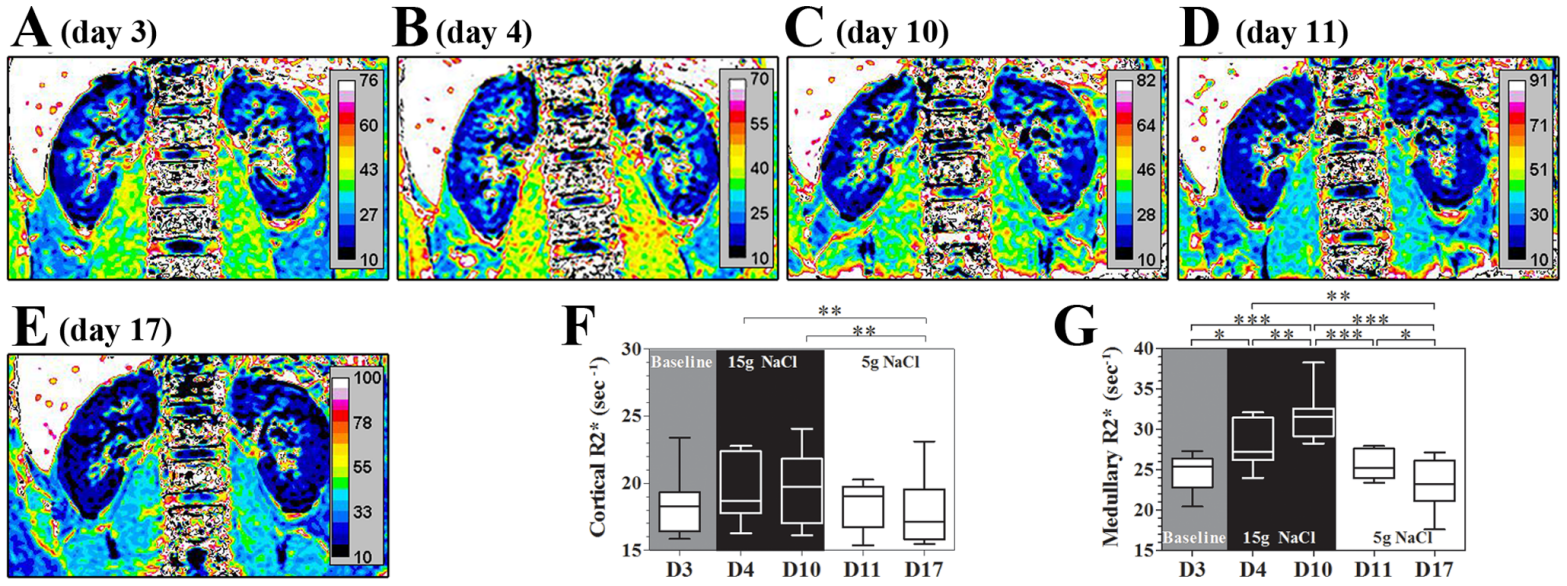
Renal blood oxygen level dependent-magnetic resonance imaging (BOLD-MRI) during dietary intervention. Figures A to E show the representative changes of BOLD-MRI images from one participant during dietary intervention on day 3, day 4, day 10, day 11 and day 17, respectively. Figures F and G show R2*signal changes in renal cortex and medulla, respectively. Statistical comparisons are derived from 11 subjects by one way repeated measures ANOVA. P*<0.05, *P*<0.01, P*<0.001. “D3” to “D17” labeled in the x-axis indicates “day 3″ to “day 17″.
